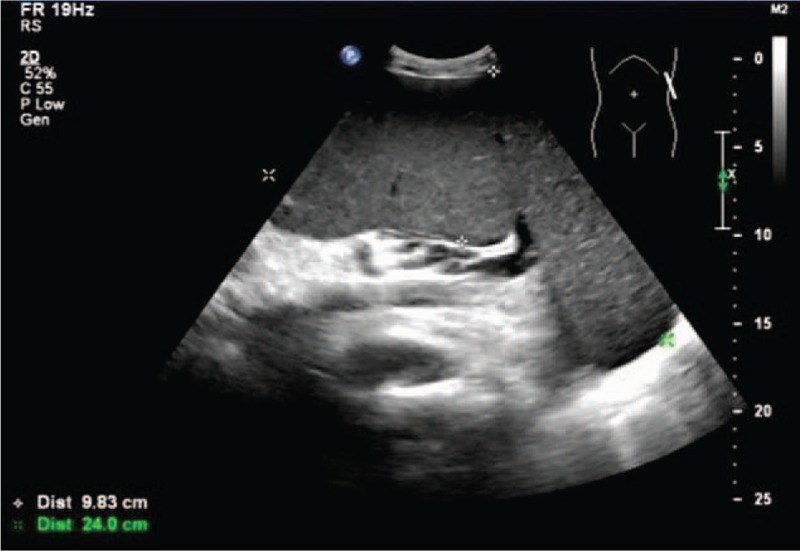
The B-mode ultrasonography of spleen.
